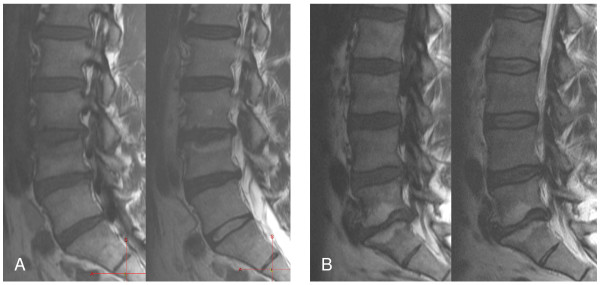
A: MR images of the lumbar spine in a 40-year-old paper mill worker demonstrate Modic type I change (low T1 and high T2 signals) at both endplates of L3-L4.B: MR images of the lumbar spine in a 49-year-old train engineer demonstrate Modic type II change (high T1 and T2 signals) at both endplates of L5-S1.
